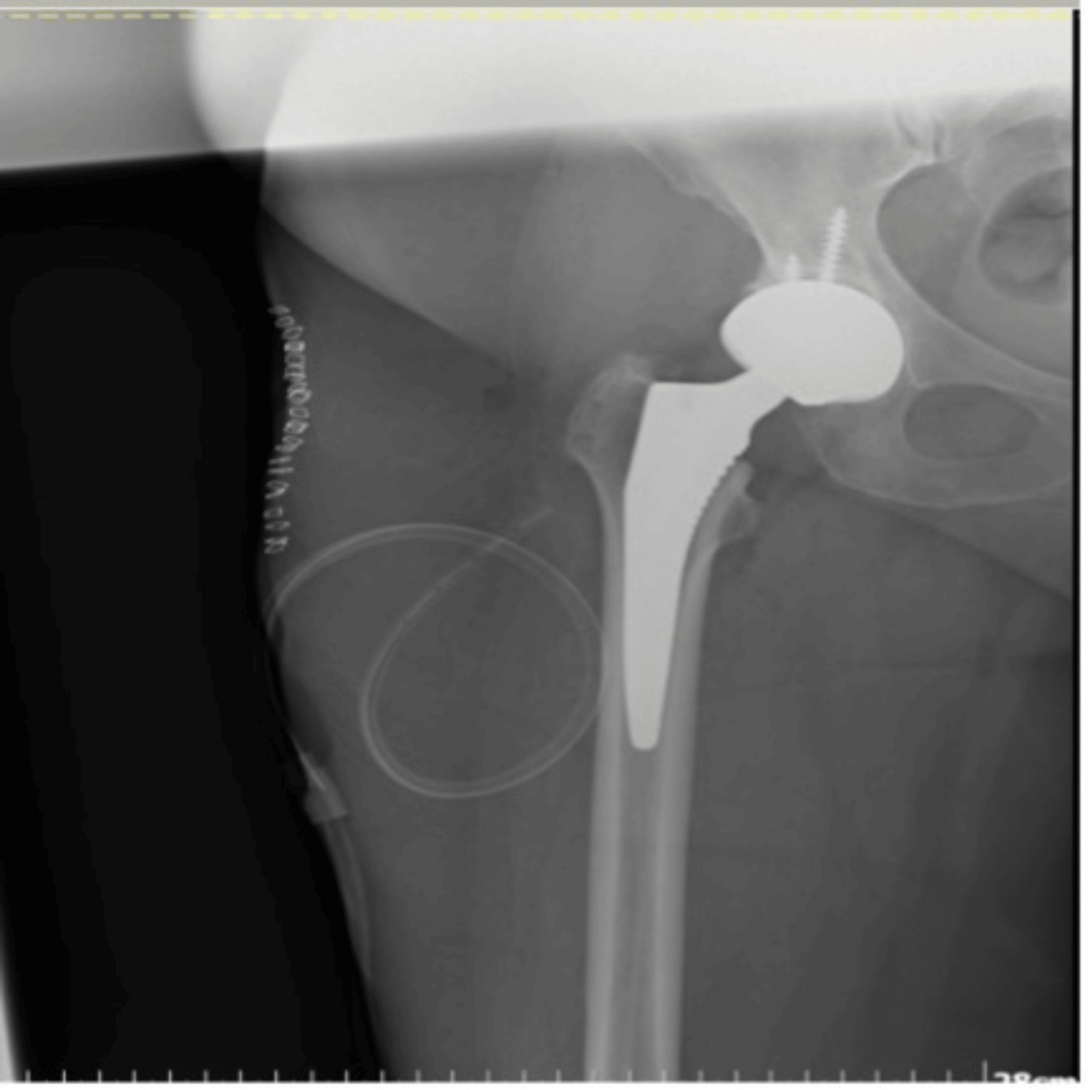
Immediate postoperative plain radiograph
